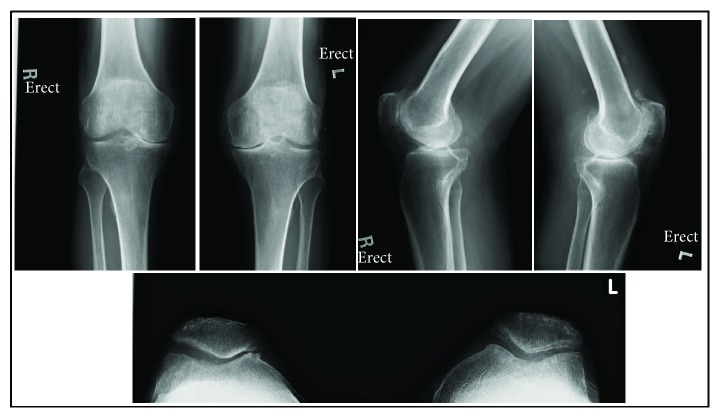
Pre-operative X-rays of the knees.
